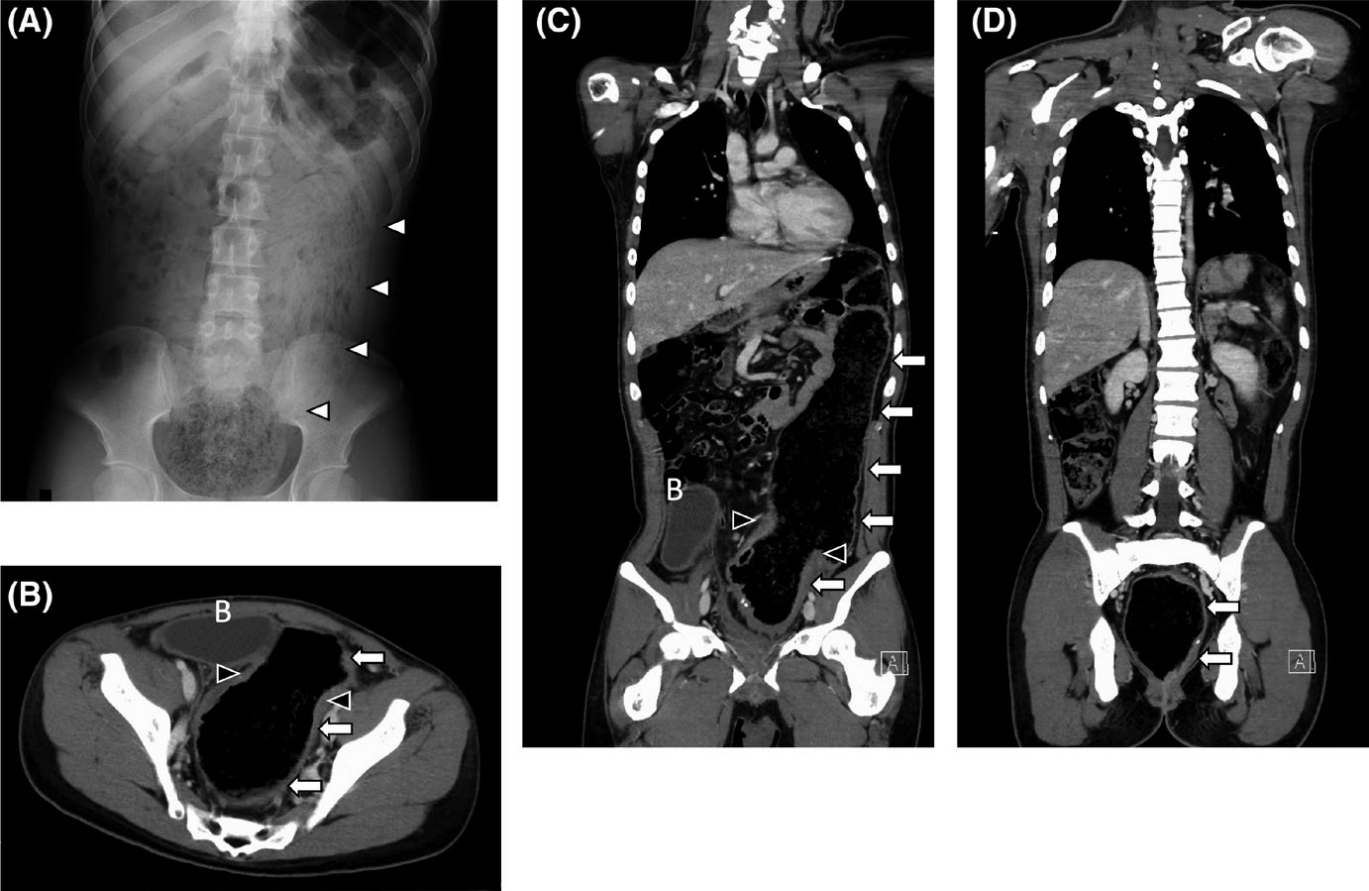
(A) Finding of abdominal X‐ray showed a stool mass from the descending colon to the rectum. Axial (B) and coronal (C, D) dislocation of abdominal enhanced computed tomography showed a highly dilated large intestine, large amounts of residue, and gas retention. White arrows; dilated intestinal tract, white arrowheads; stool mass, black arrowheads; anastomotic site, B; bladder
